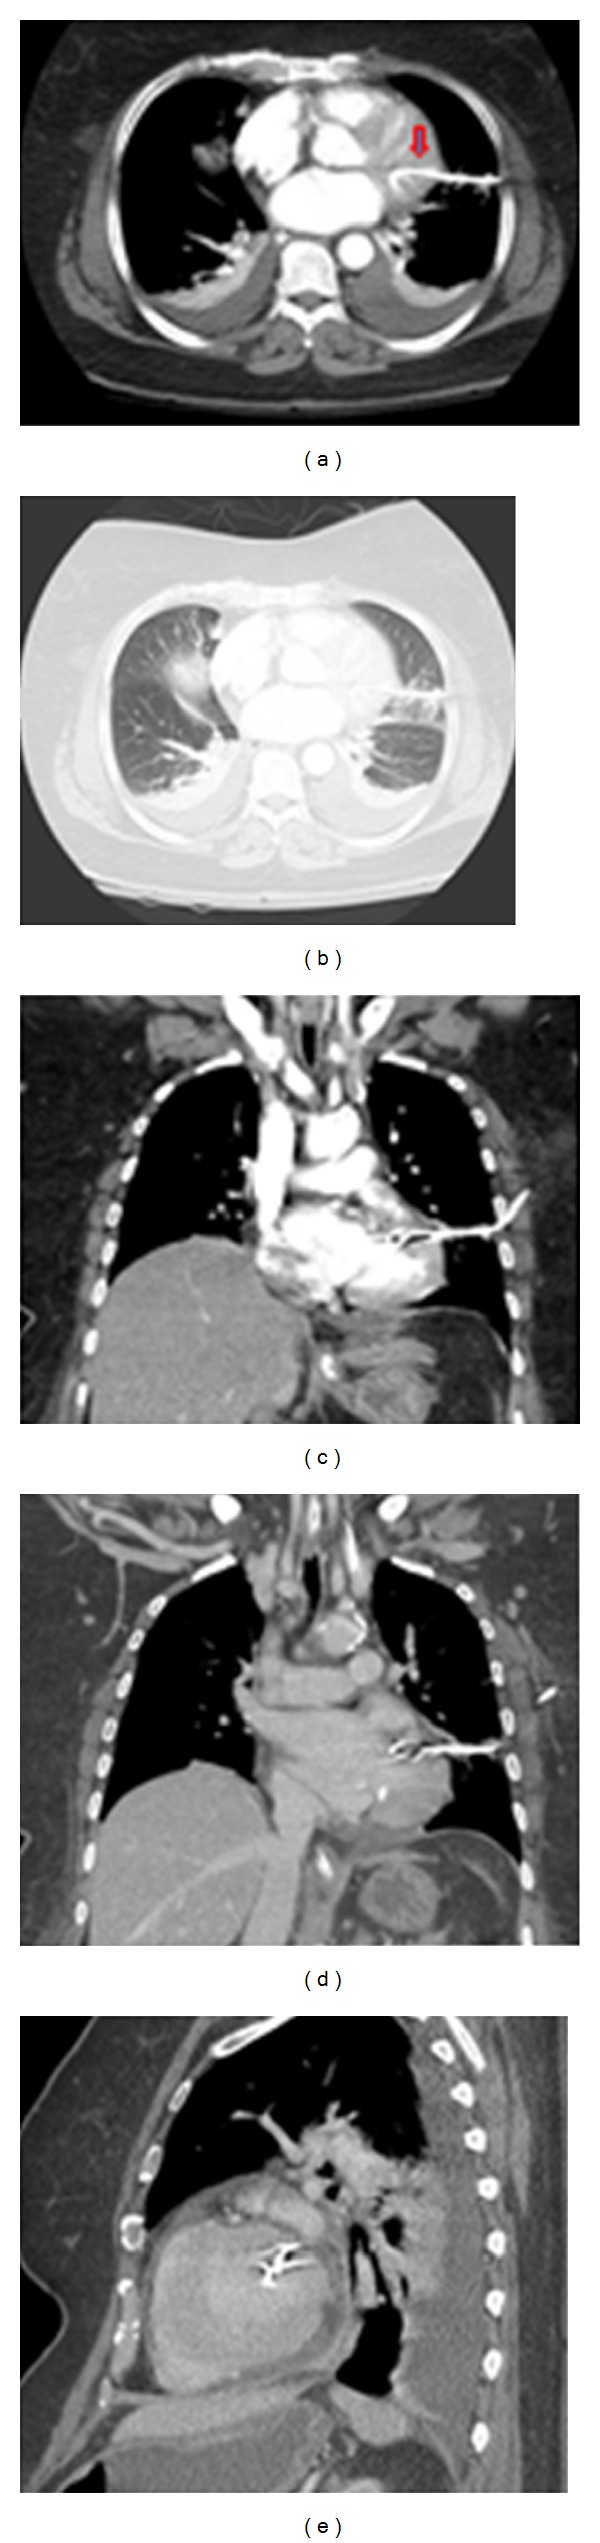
It shows a portable chest radiograph on an intensive care patient that developed pleural effusions. A chest drain was placed to drain the left-sided pleural effusion. Bright red blood was obtained from the tube. A CT scan (Figures 28 and 30) obtained immediately revealed the tip of the catheter had entered the left ventricle. The patient was immediately taken for cardiac surgery and the tube was removed without complications.
